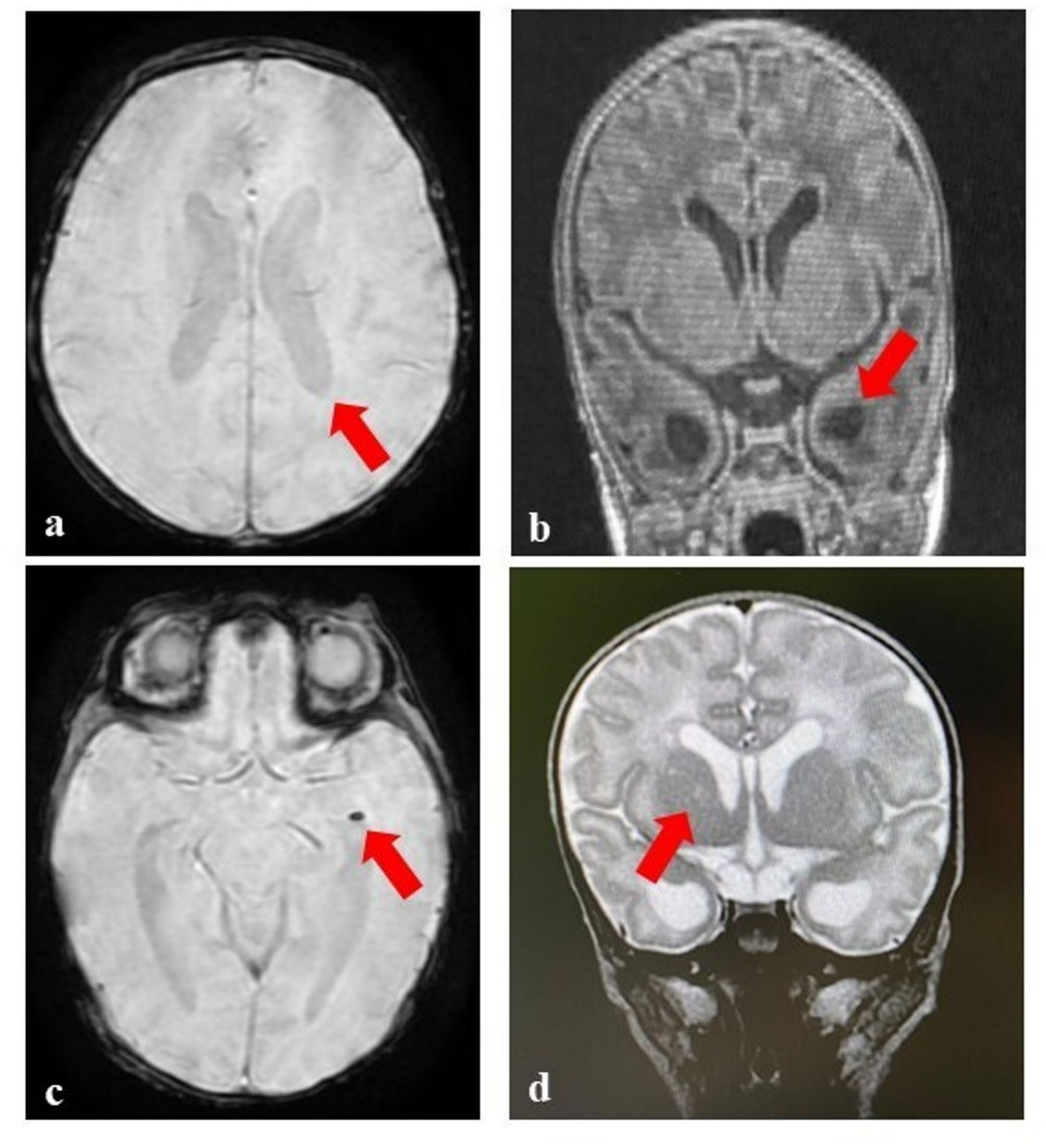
Brain MRI. a Lateral ventricles dilation; b cystic lesions within the ependyma of the temporal horns; c millimetric calcification in the left ventricular ependyma; d lacunar lesion within the right thalamus. All findings are indicated by arrows
